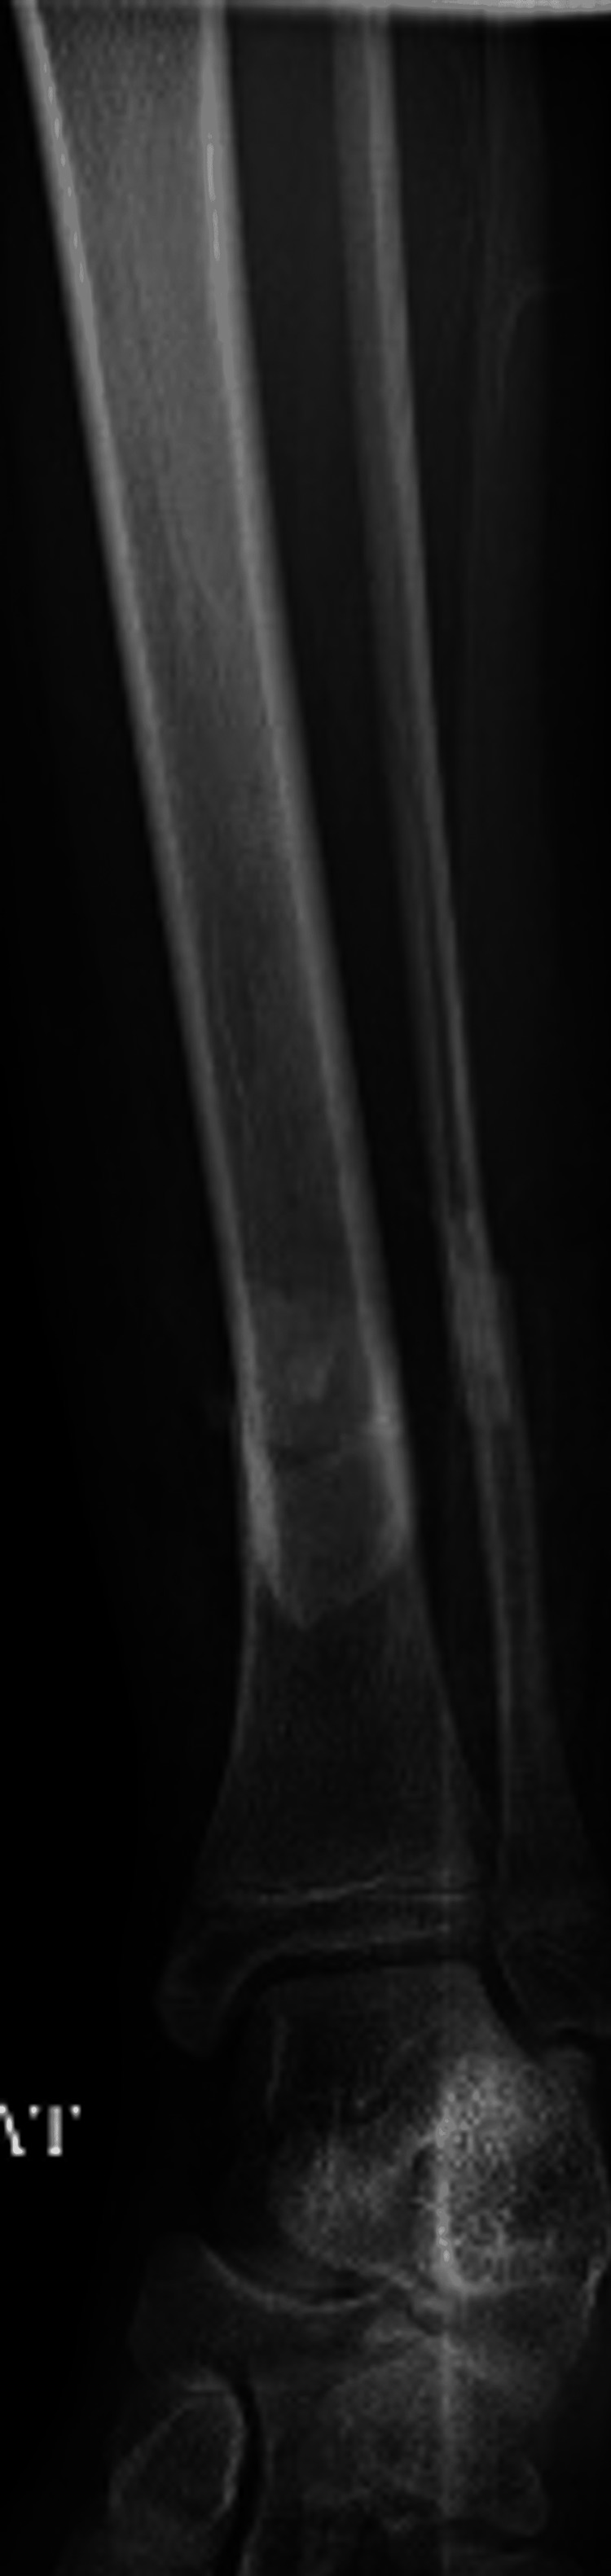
Anterior-posterior tibia-fibula radiographs of initial open tibia/fibula shaft fractures.
